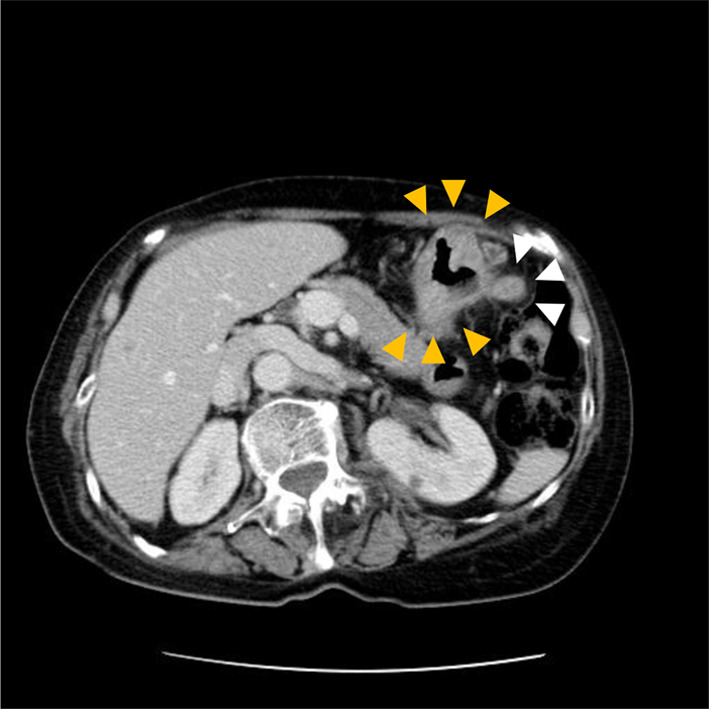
Abdominal CT. There is irregular wall thickening with a contrast effect on the posterior wall of the lower stomach (yellow arrow). Multiple enlarged lymph nodes are observed on the greater curvature of the stomach (white arrow). No obvious distant metastasis is observed
